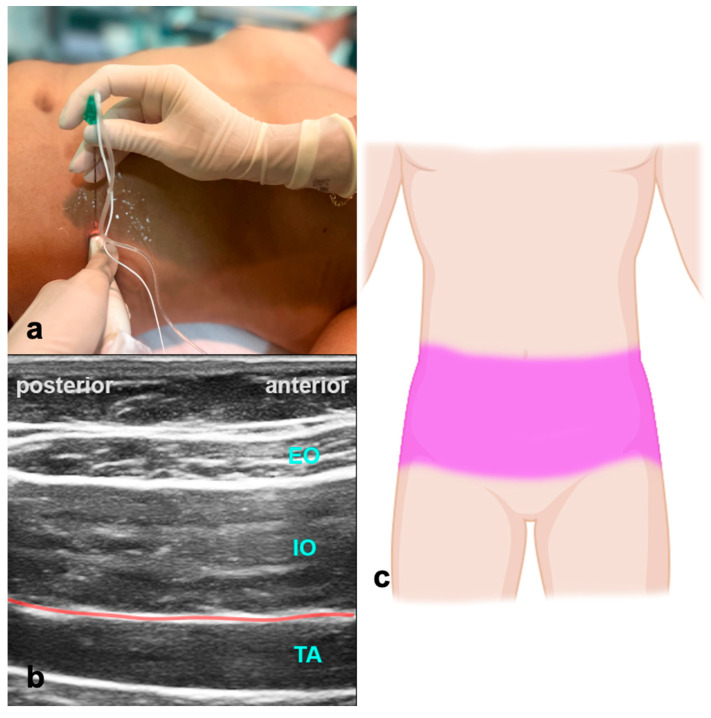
Transversus abdominis plane block. (a) Transducer positioning for TAPB. (b) Ultrasound anatomy of TAPB: EO—external oblique muscle; IO—internal oblique muscle; TA—transverse abdominal muscle; red line—target space for TAPB. (c) Spread of TAPB if applied bilaterally.
